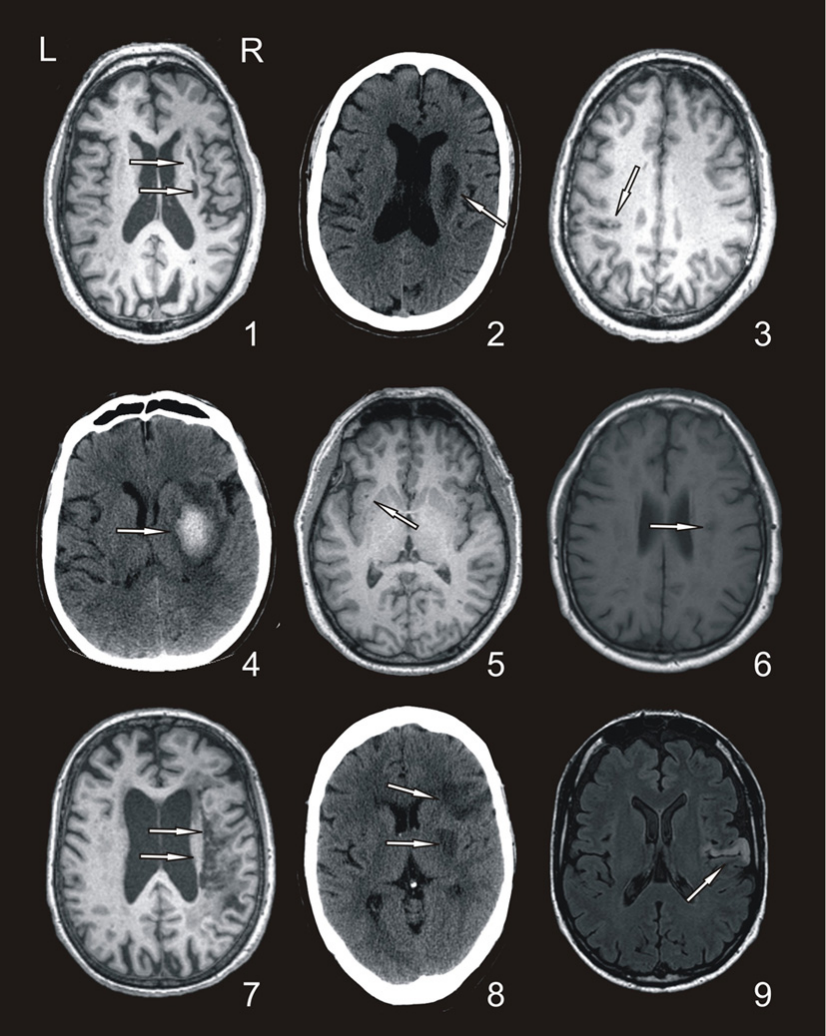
Representative axial Magnetic Resonance and Computed Tomography images of the patients. Arrows show the approximate location of each lesion (patient codes are according to the order in Table 1).
